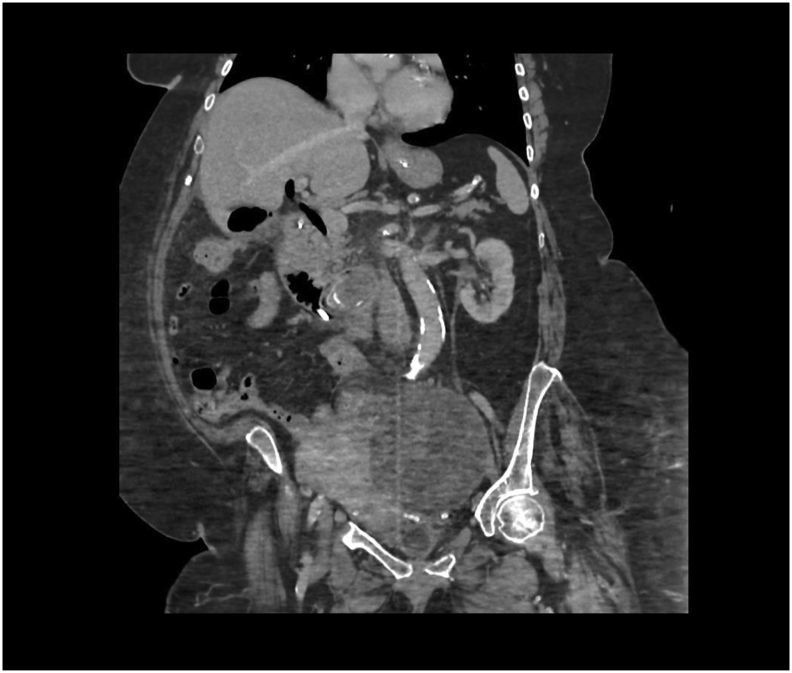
CT abdomen pelvis on admission. Coronal slice of CT abdomen pelvis with visible ectopic gallstone and cholechoduodenal fistula.
